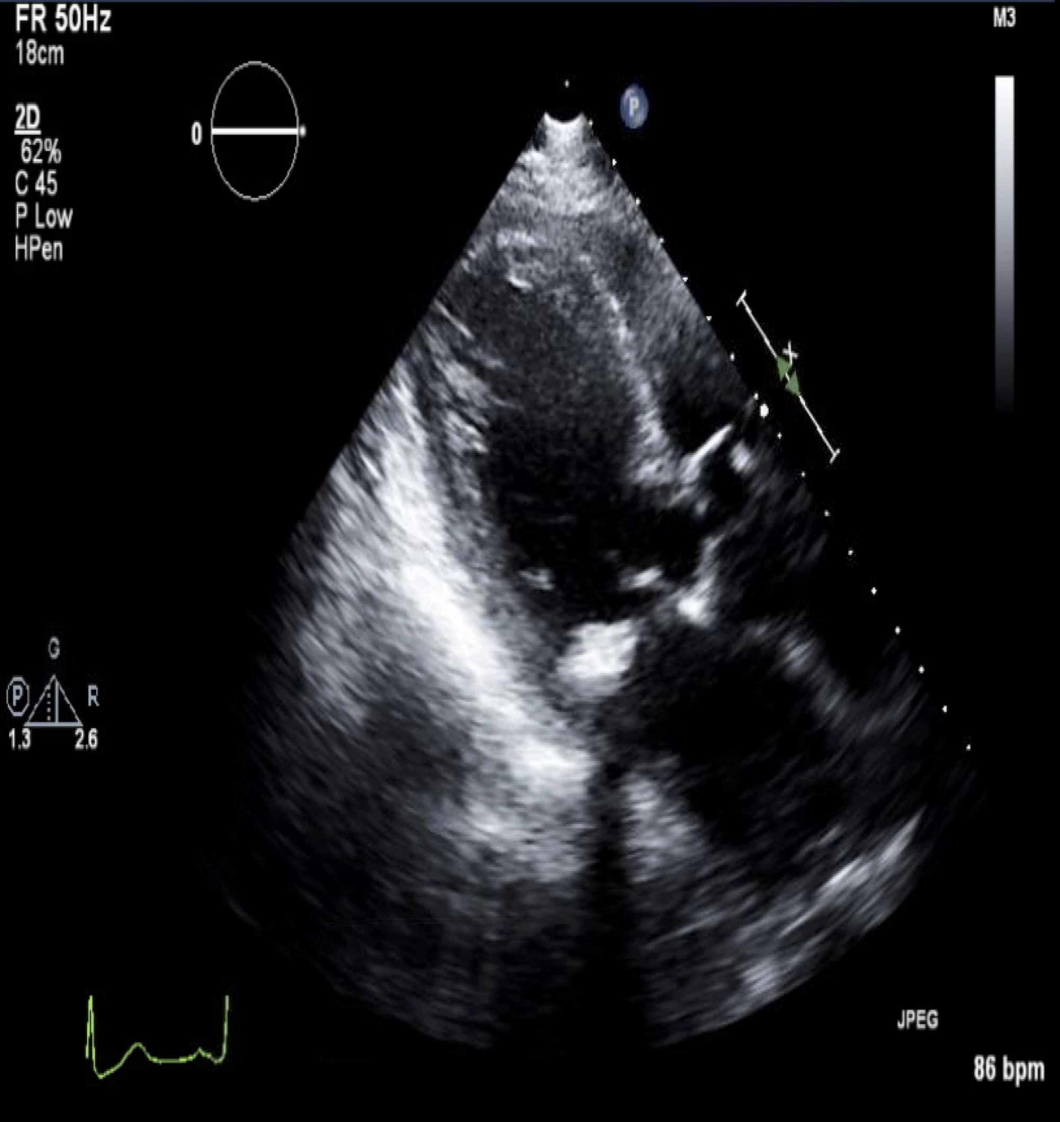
TEE showing left ventricle during diastole TEE: transesophageal echocardiography
